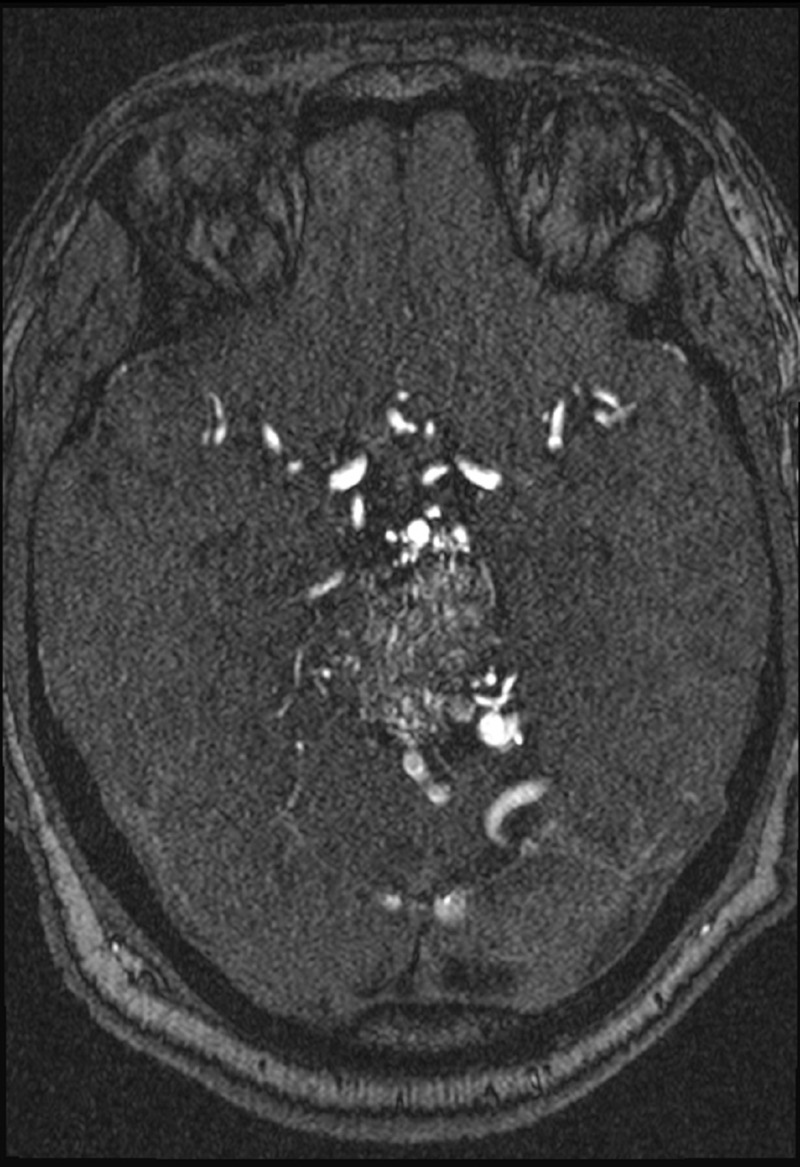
MRI head with contrast, axial section
